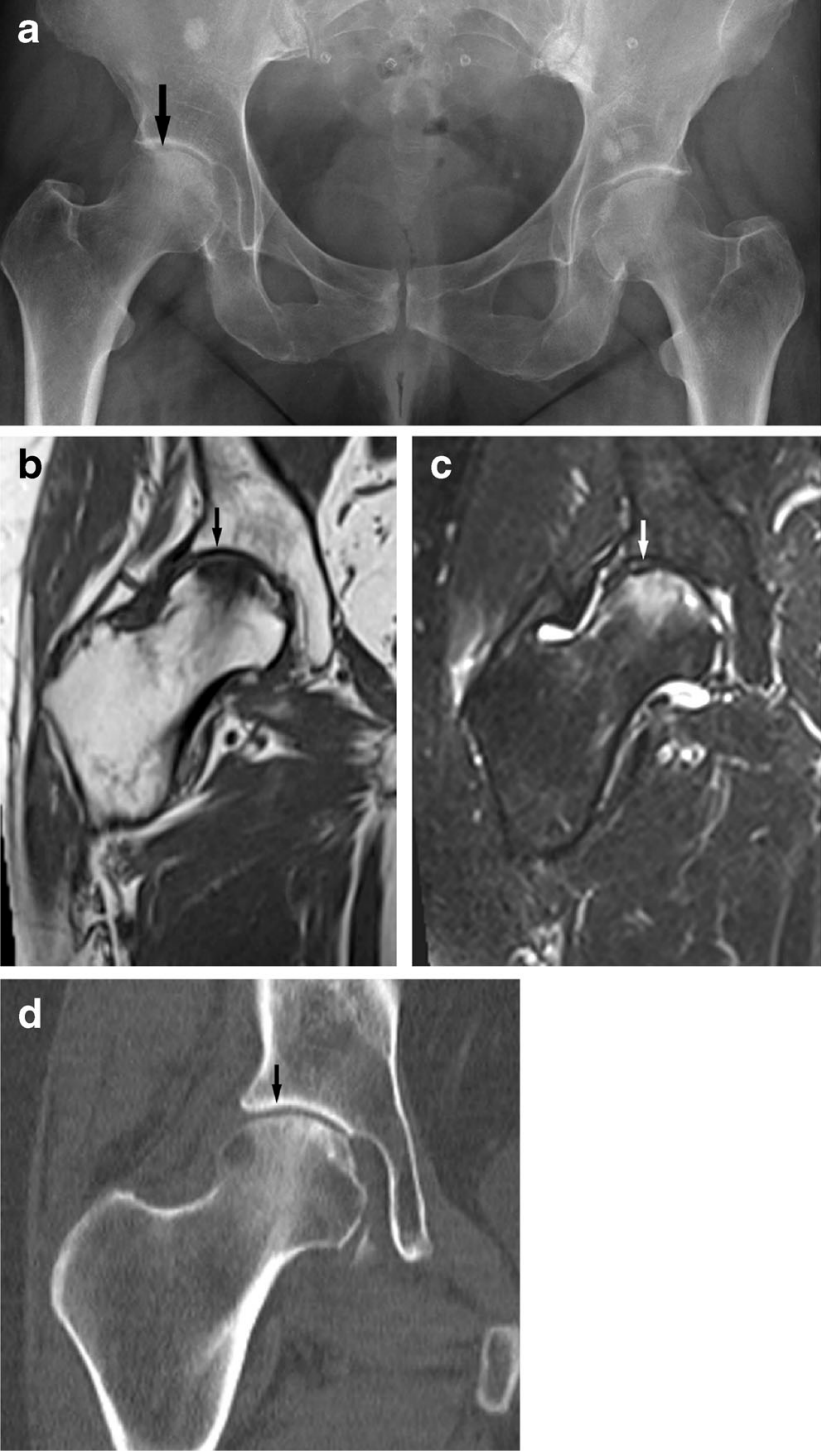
A 60-year-old female presenting with groin pain. An AP radiograph of the pelvis (a) shows subtle subchondral flattening of the femoral head suspicious for a stress (insufficiency) fracture (see arrow). Multiple bone islands are noted. Sclerosis and irregularity of the symphysis pubis again suggest bone stress. On radiography alone avascular necrosis also has to be considered as a cause of subchondral flattening; however the femoral head infraction is confirmed with MRI [coronal T1w (b) and T2-FS (c) images] (see arrows) and avascular necrosis (avn) is effectively excluded. This is confirmed with CT imaging (d). These lesions need specialist attention to prevent deterioration to rapidly destructive OA
